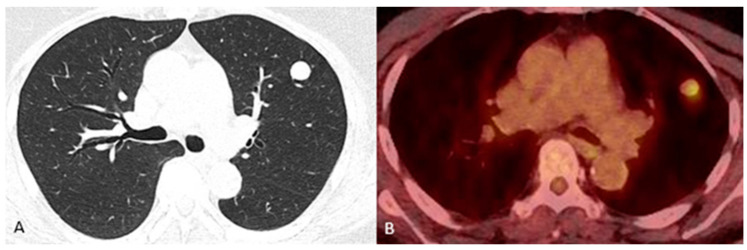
(A) Chest CT without contrast. (B) Fused PET and CT of an 84-year-old female diagnosed with a primary pulmonary meningioma. CT = computed tomography. PET = positron emission tomography.
